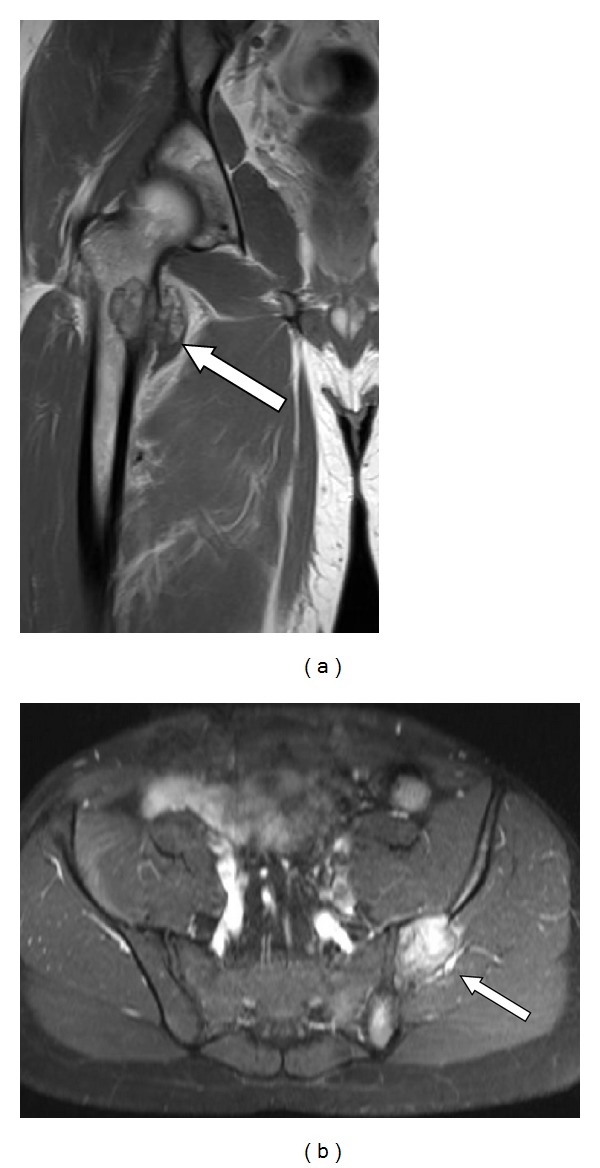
Magnetic resonance imaging: (a) coronal image of the right proximal femur shows the soft tissue involvement (arrow) of the lesser trochanteric region, and (b) axial image of the pelvis demonstrates the soft tissue involvement of the right gluteal region (arrow) nearby the iliac wing and the sacroiliac joint.
